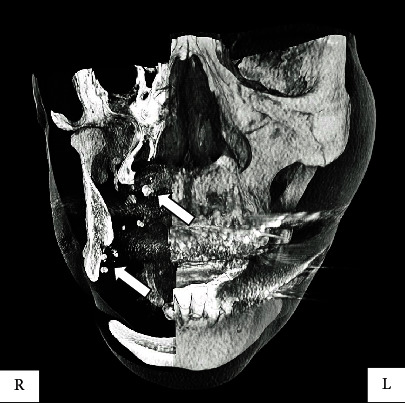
Computed tomography image showing numerous calcifications of unequal size in the medial portion of the right mandible. Circular hard tissue is evident (arrow).
